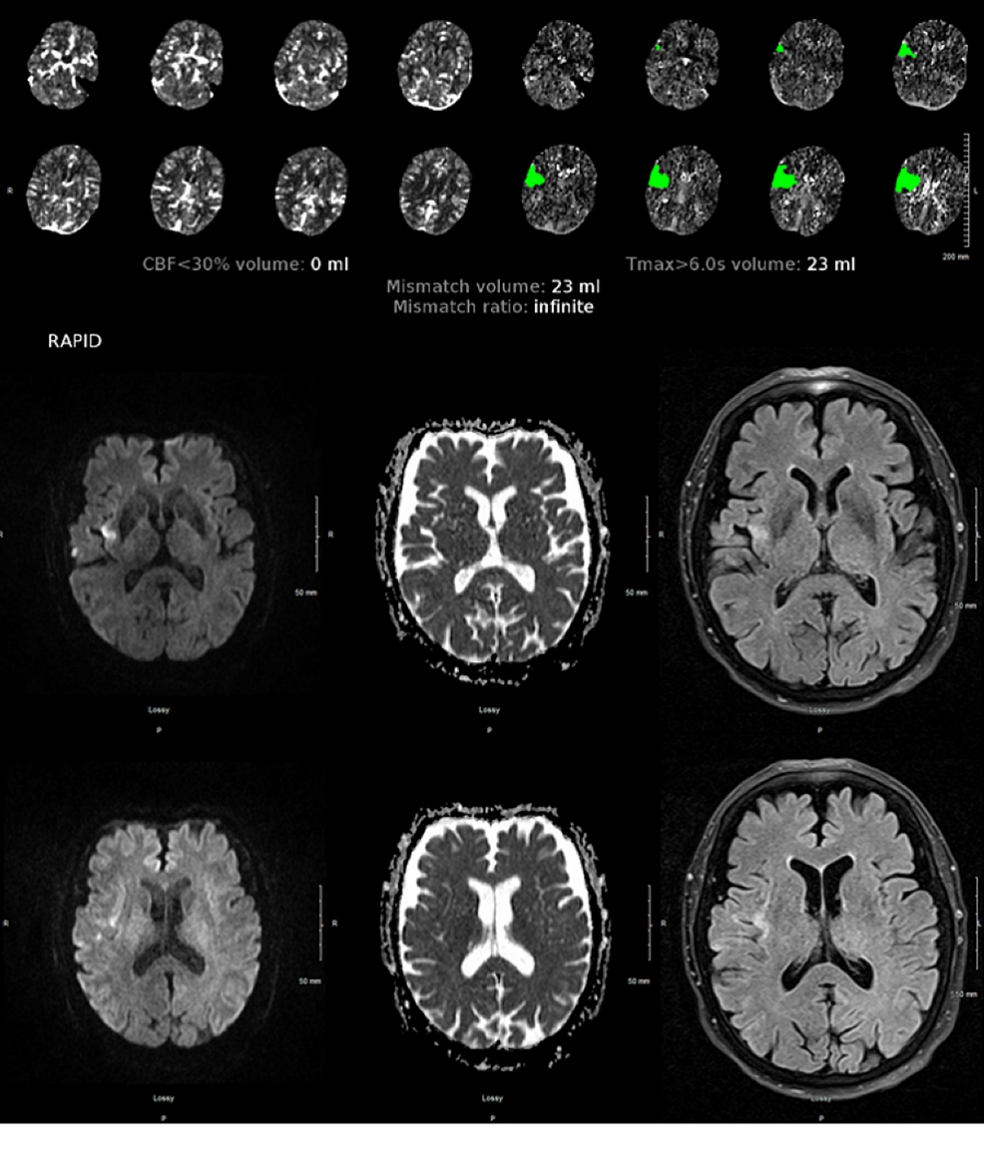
Scans of Patient B Patient B’s initial CT perfusion scan (above) showed a moderate-sized 23 ml penumbra in a right MCA branch territory. However, MRI brain (below) revealed a final infarct volume of only 0.5 ml.
